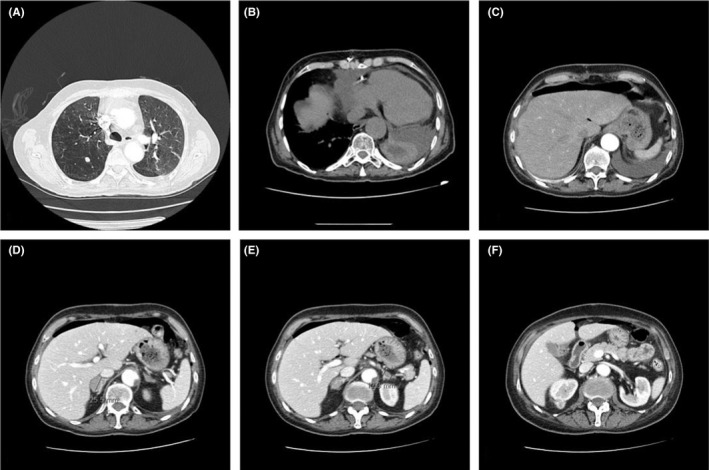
CT scan: (A) calcific nodule in the right lung; (B) significant pericardial effusion; (C) free peritoneal air; (D, E) an adenoma‐like lesion in the right adrenal gland and a similar one in the left adrenal gland; and (F) exophytic lesion of the upper pole of the right kidney
